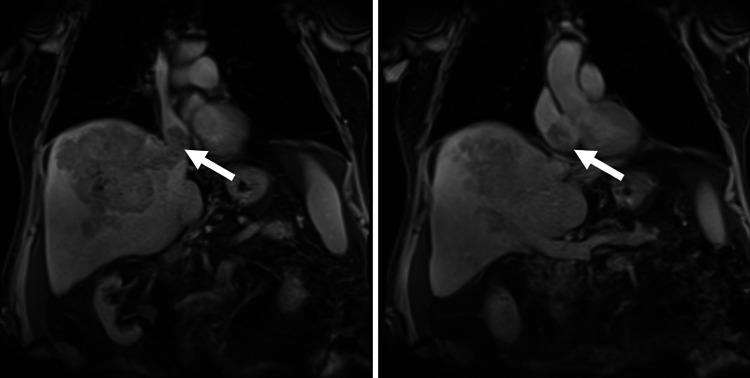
Magnetic resonance imaging (MRI) of the tumor thrombus invading into the right atrium of the heart.
